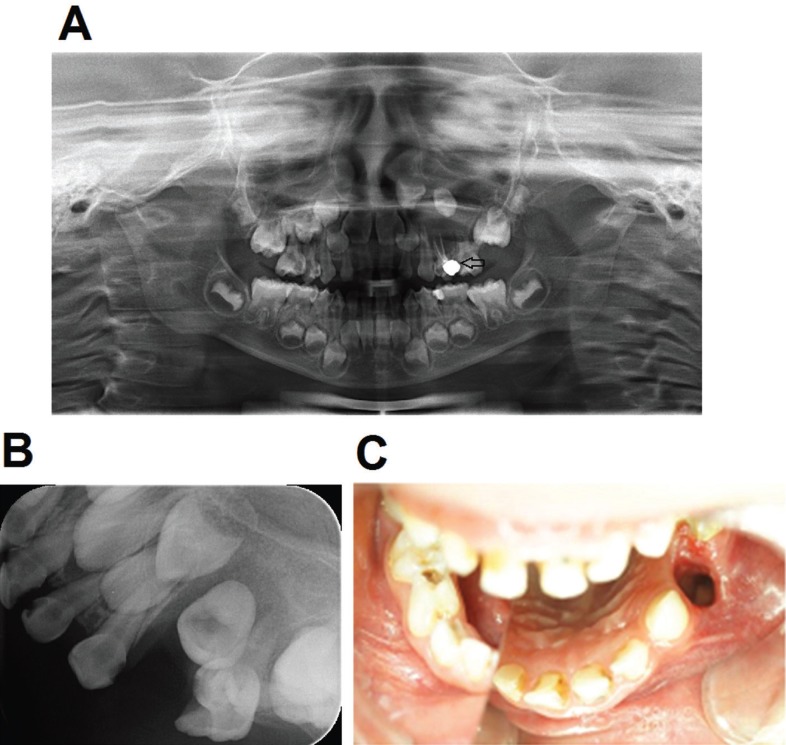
Preoperative and postoperative examination. The abnormal radiolucency also displaced upwards the bud of the left maxillary first premolar (A). The periapical radiograph showed a reduction in the size of the radiolucent lesion and a spontaneous eruption of T24 (B); and the marsupialization, the crown of T24 could be seen through the cyst window (C) 3 months after the procedure.
